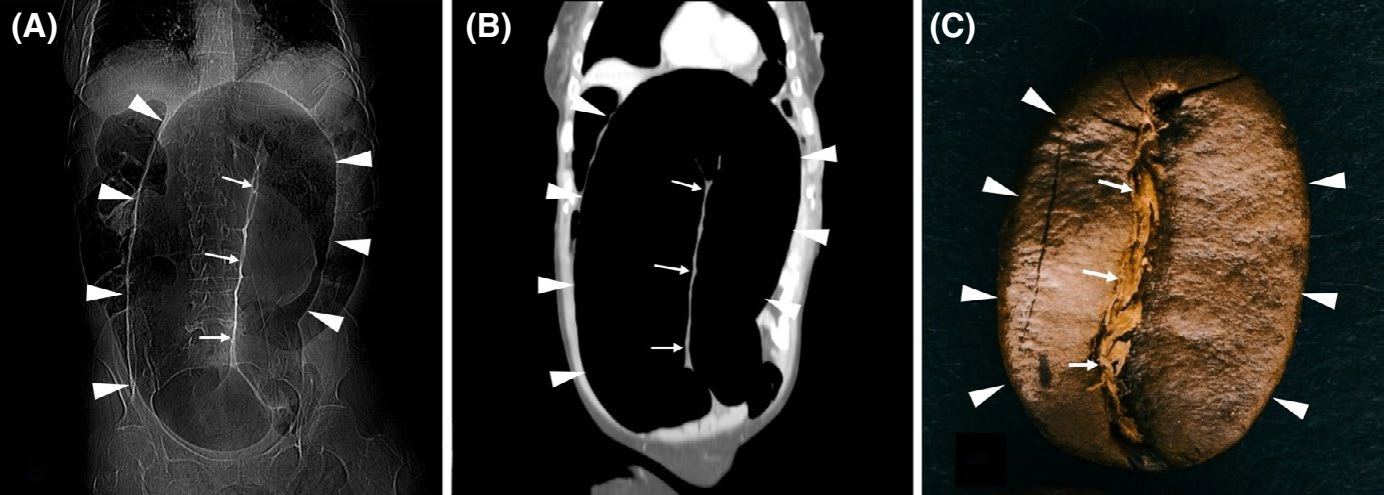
A computed tomography scout view (A) and a coronal image (B) demonstrating the picture of a coffee bean (C). The two parts of the coffee bean represent the gas‐filled segments of the dilated bowel (arrowheads), whereas the central cleft of the coffee bean represents the double thickness of opposed bowel walls (arrows)
